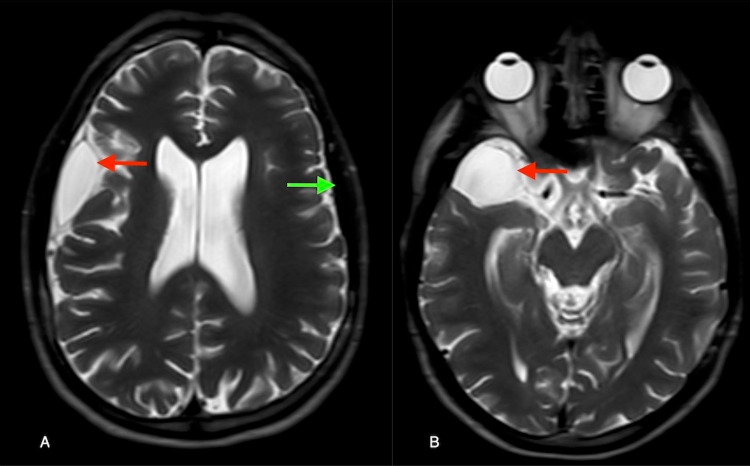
Axial T2 MRI brain at the level of the lateral ventricles (A) and the midbrain (B) demonstrating interval resolution of acute subdural hematoma (SDH) (green arrow) and chronic SDH (red arrow) and arachnoid cyst (red arrow) at 3.5 year follow-up.
